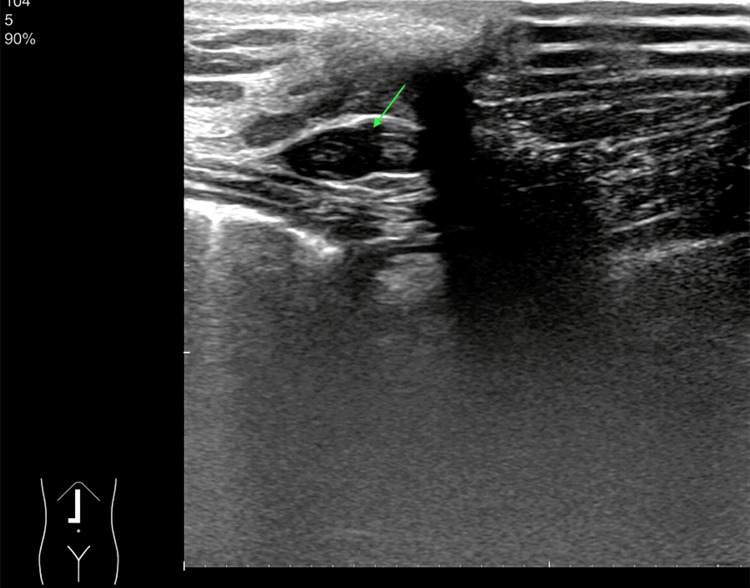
Ultrasound image showing a well-defined oval solid-cystic hypoechoic subcutaneous nodule (arrow) measuring 8.9 × 5 × 5.3 mm in the immediate supraumbilical region along the midline of the abdomen.
